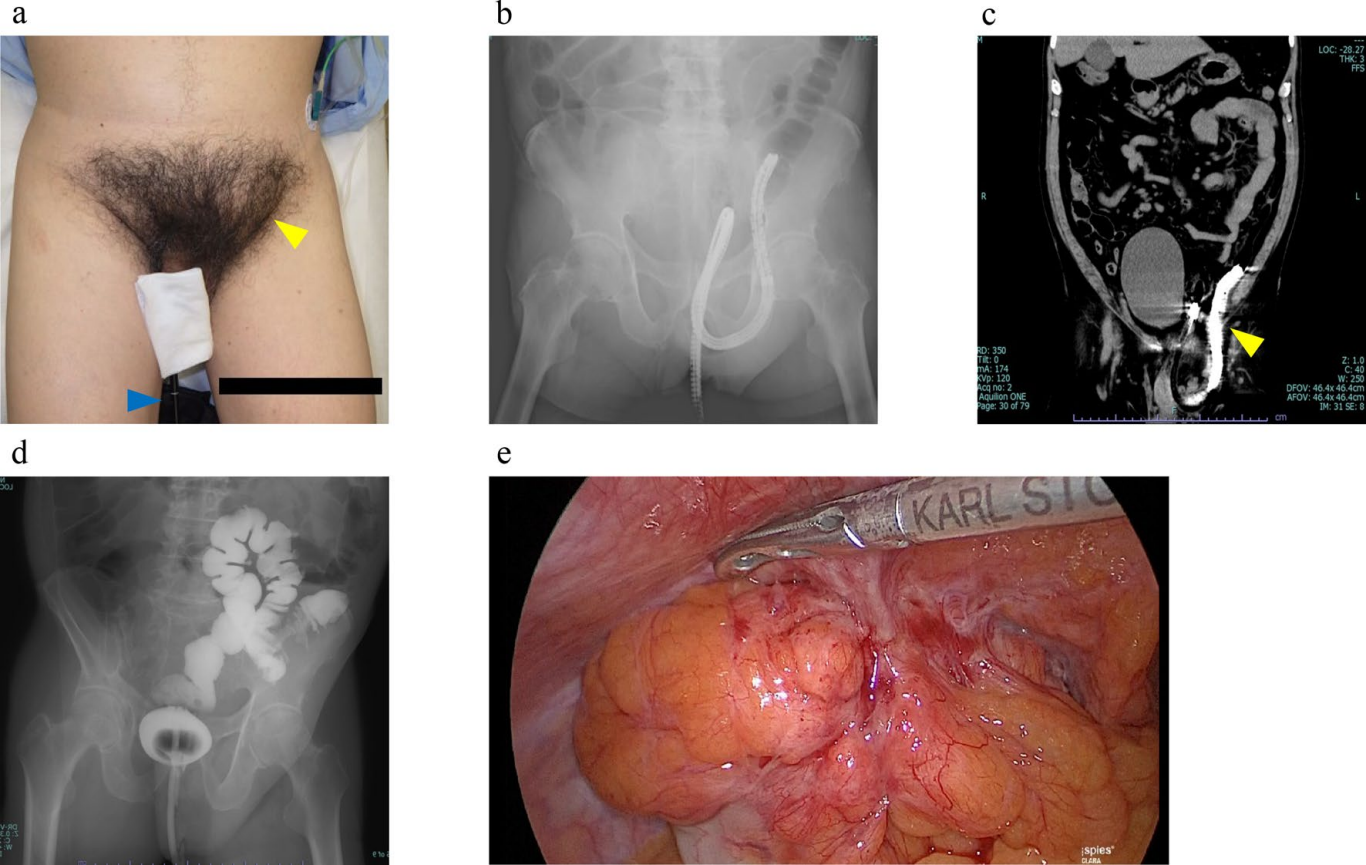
Representative images from abdominal examinations. a Colonoscope remained in situ via the anus, and the patient’s left inguinal region was swollen. The blue arrowhead indicates colonoscope remained in situ via the anus. The yellow arrowhead indicates left inguinal bulge. b Abdominal radiography showed the colonoscope incarcerated within the left inguinal hernia. c Computed tomography scans of the abdomen revealed the presence of the colonoscope. The yellow arrowhead indicates colonoscope within the left inguinal hernia. d Fluoroscopic examination using a gastrografin enema showed no signs of perforation. e Intraoperative image showing a large direct inguinal hernia, incarceration of the sigmoid colon within the hernia sac, and extensive adhesions around the hernia site
